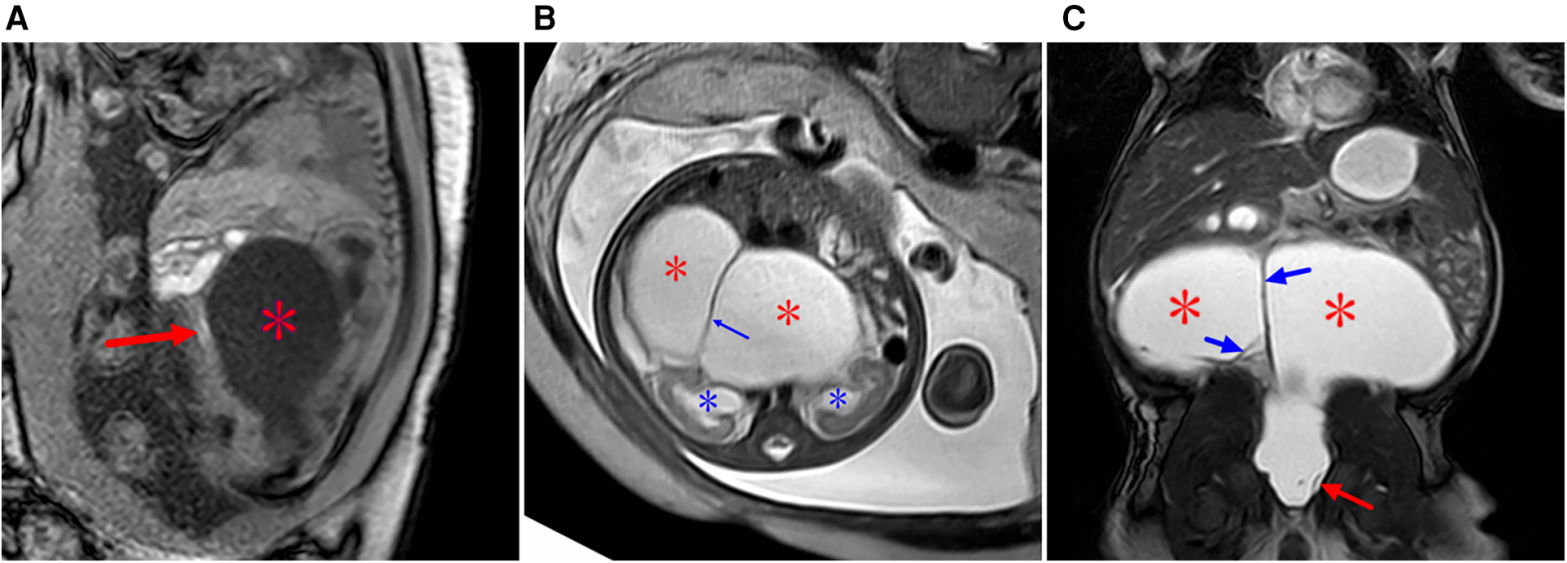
Type IV sacrococcygeal teratoma (misdiagnosed as cloacal malformation on fetal MRI) at 33 weeks of gestation. (A) On T1WI, the ellipsoidal low signal mass (red star) is seen in front of the sacral coccyx, and a strip of high signal (suspected rectum) is seen faintly in front of the lesion (red arrow). (B) The lesion is seen posteriorly in the abdominal pelvis, showing high signal intensity on T2WI, with linear separations (blue arrows) showing the left and right halves (red stars), and bilateral hydronephrosis of the fetus (blue asterisk). (C) On T2WI MRI after birth, the sacrococcygeal mass (red star) is seen with multiple separations (blue arrows) originating in the sacral caudal mass (red arrows).
